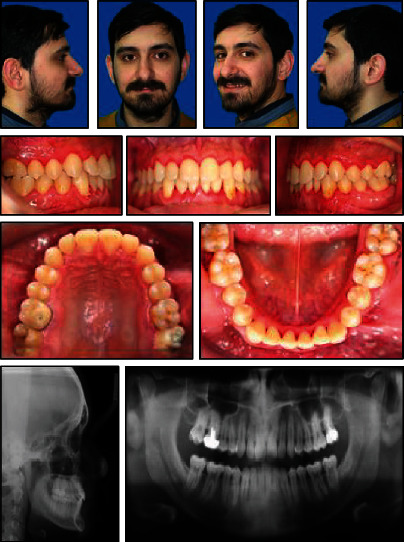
Facial and intraoral photographs, lateral and panoramic X-rays after 10-year retention.
